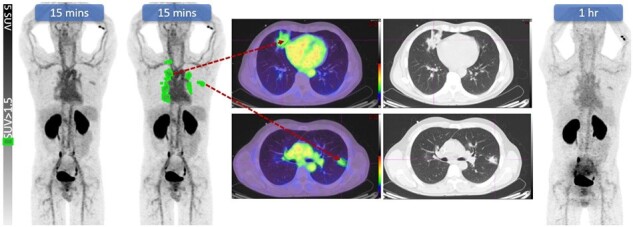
68Ga-TAFC-PET/CT in a patient with leukemia and probable pulmonary A. fumigatus IAI. Specific infective uptake of known pulmonary lesions at around blood pool level intensity (SUVmax 3.5, SUVmean 2.3). There was minimal liver and marrow uptake, with progressive washout to 60 minutes.
